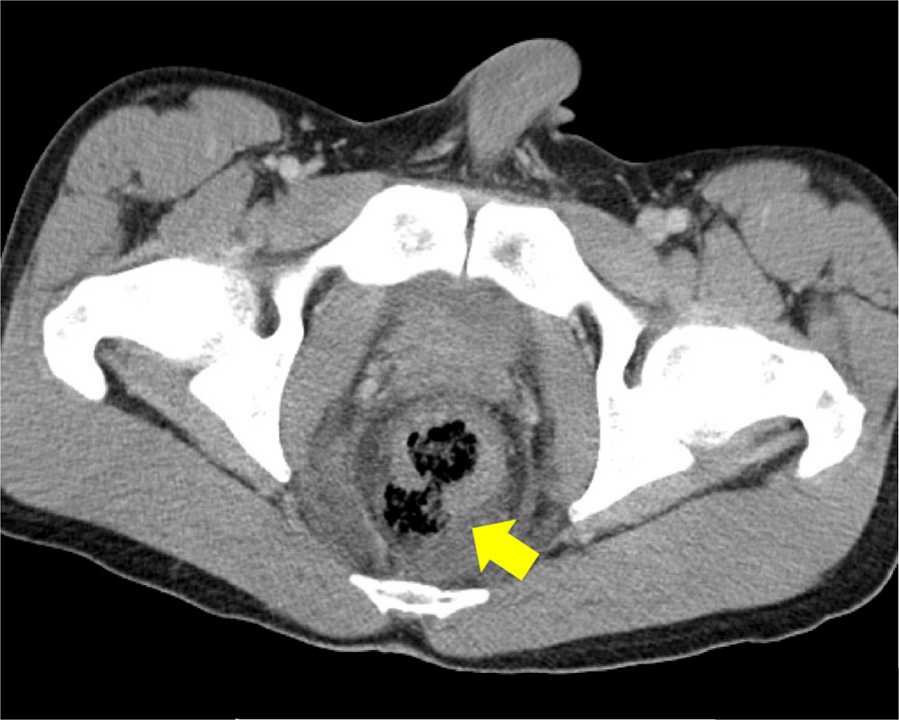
Abdominal CT scan revealed full-layer perforation in the posterior rectal wall and leakage of feces into the extraperitoneal space (yellow arrow)
